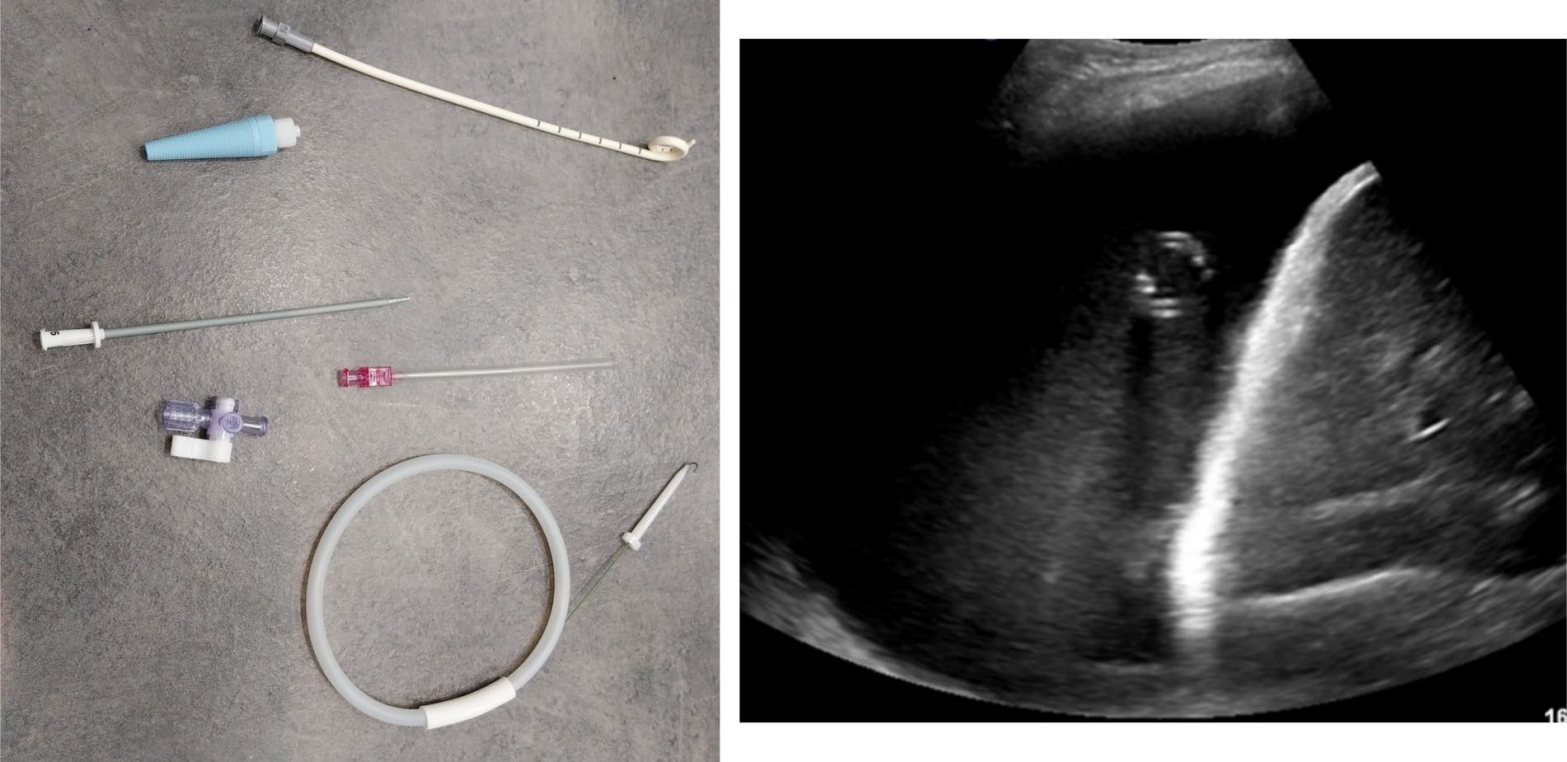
Small bore chest drain placement technique with ultrasound. Patients were positioned appropriately, and a detailed ultrasound examination was conducted to identify the optimal insertion site. Local anesthetic was administered at the chosen site. Under ultrasound guidance, a needle was advanced into the pleural space and a guidewire was inserted through the needle. Then, a dilator was used over the guidewire to prepare the tract. The small-bore chest drain was advanced over the guidewire into the pleural space. Correct placement was confirmed by ultrasound imaging and, where indicated, chest radiography
